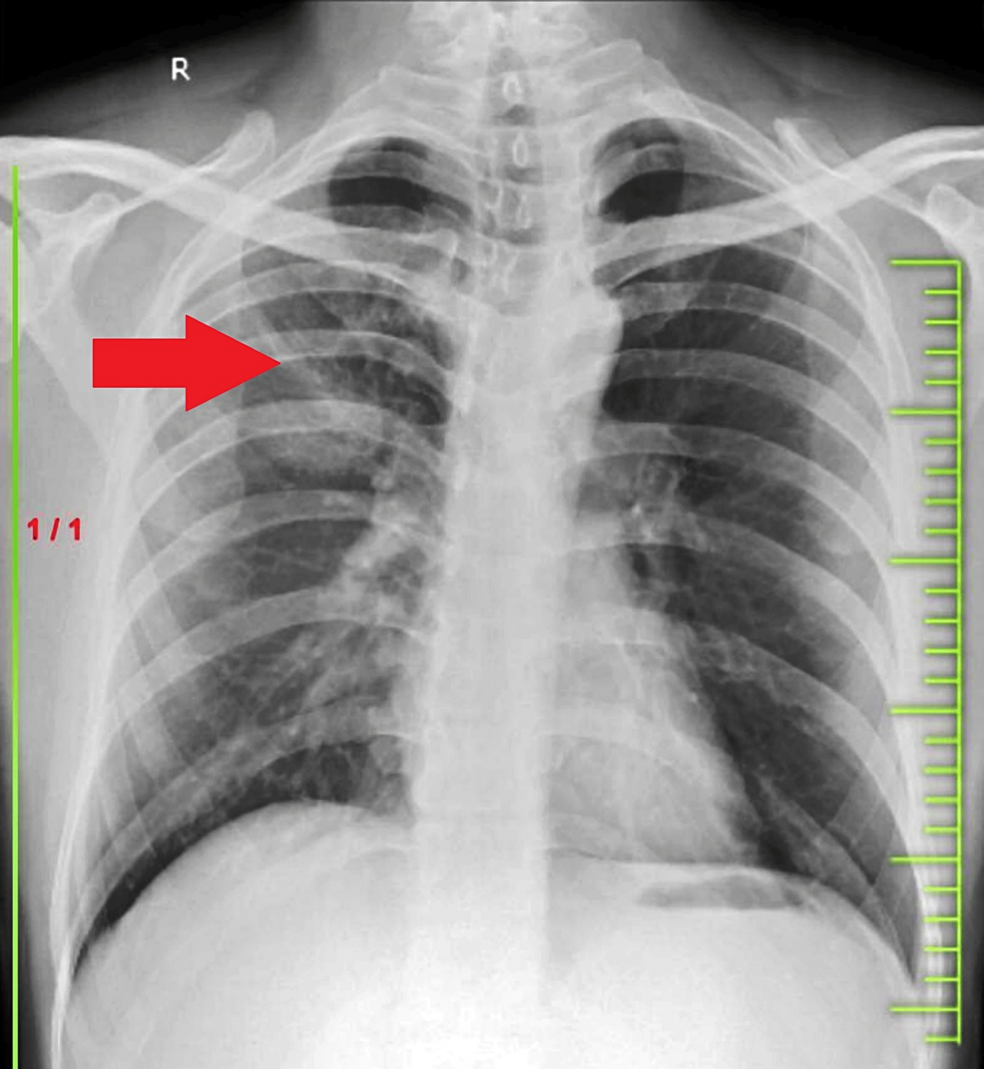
Chest radiography showing homogeneous rounded opacity occupying the right upper zone (posterior segment)
